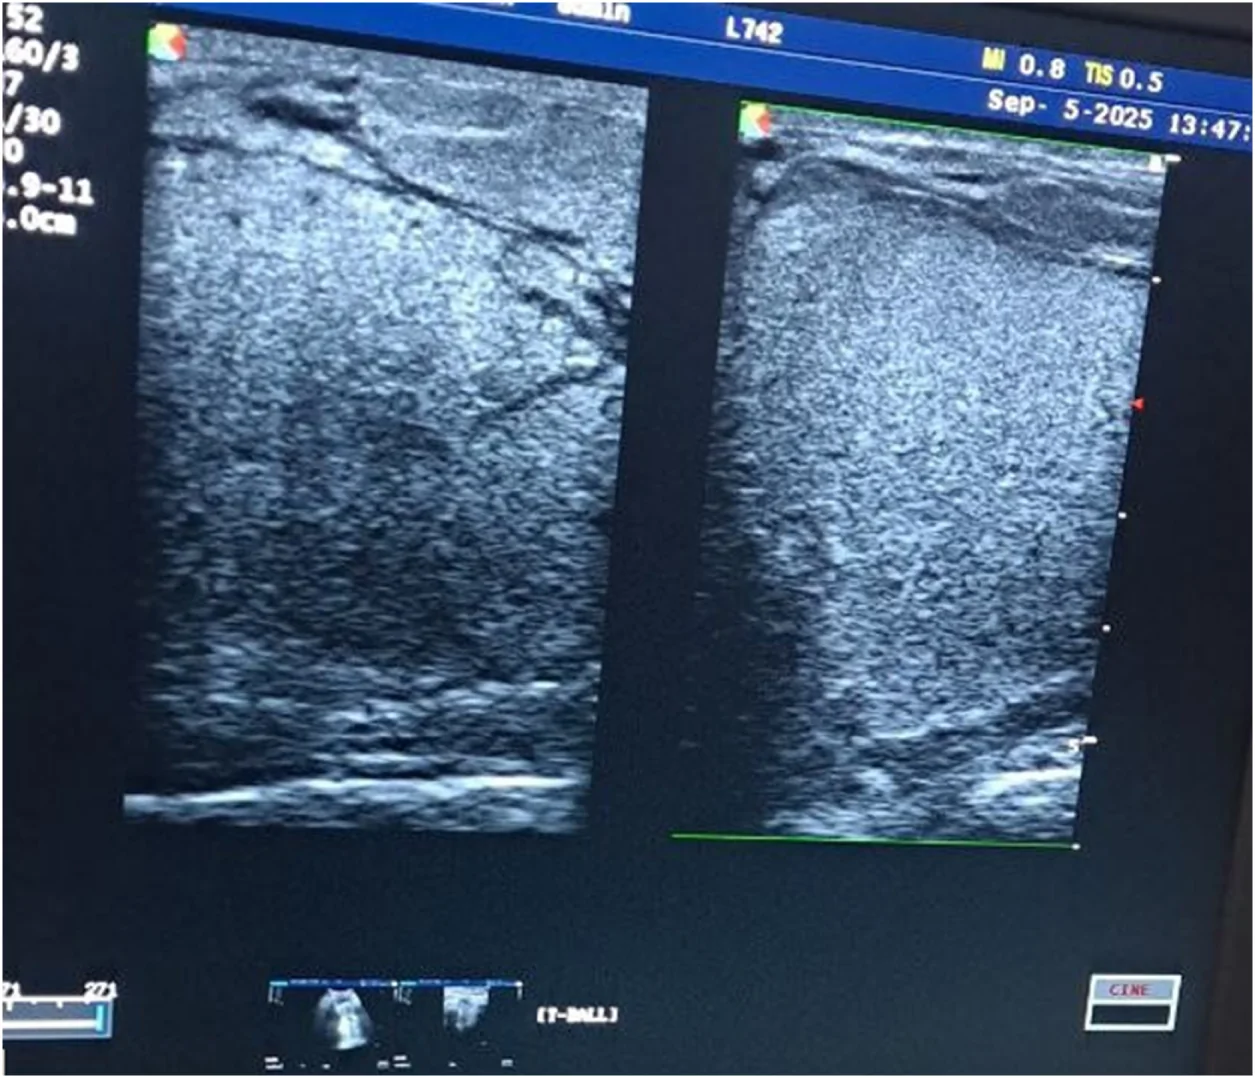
Ultrasound image showing a large, loculated, hypoechoic cystic lesion with regular margins, suggestive of a galactocele.
